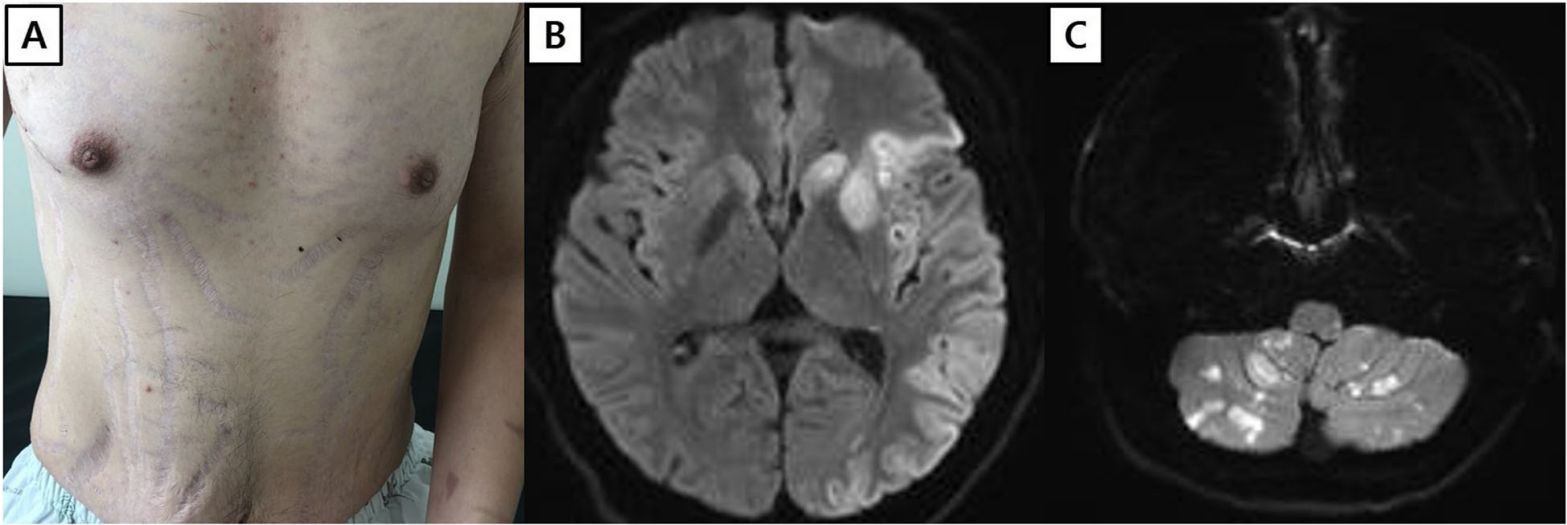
The torso of the patient shows skin striae due to Cushing’s syndrome (a). Magnetic resonance images show acute infarction on the area of left middle cerebral artery (b) and both cerebellum (c)
